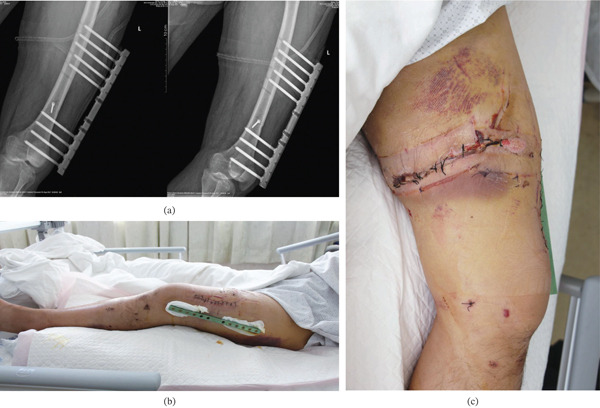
(a) Anteroposterior radiograph on postoperative Day 3 demonstrating anatomical reduction and stable fixation with the modified external fixation device and lag screws. (b, c) The incision and external fixator after VSD removal at 3 days postoperatively; no significant pin tract reaction was observed.
